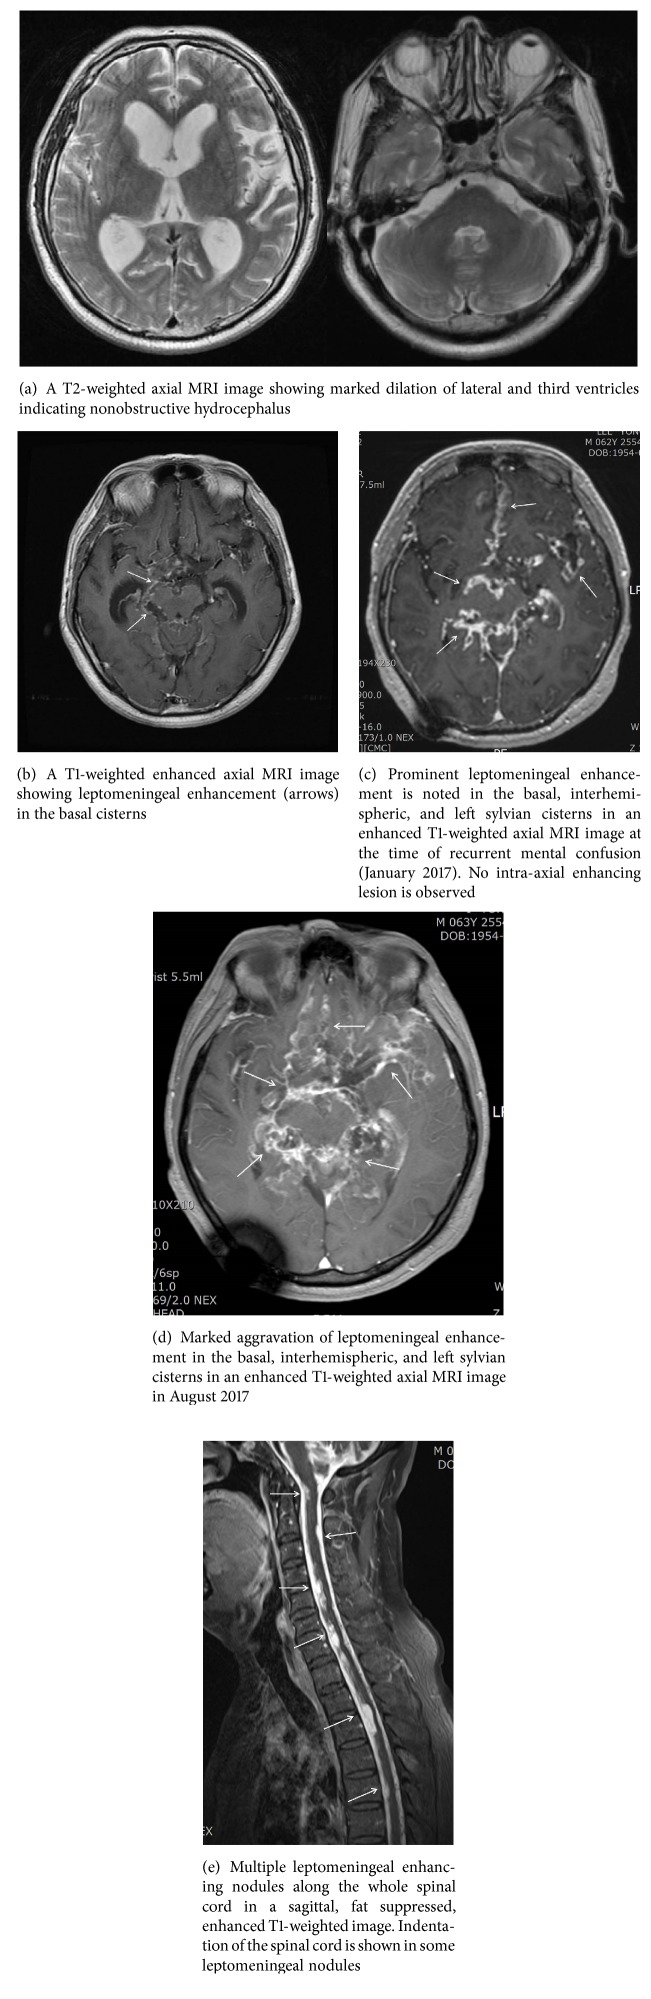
Magnetic resonance imaging (MRI) findings of communicating hydrocephalus and leptomeningeal enhancement in the basal cistern at the time of initial manifestation of mental confusion (2012).
